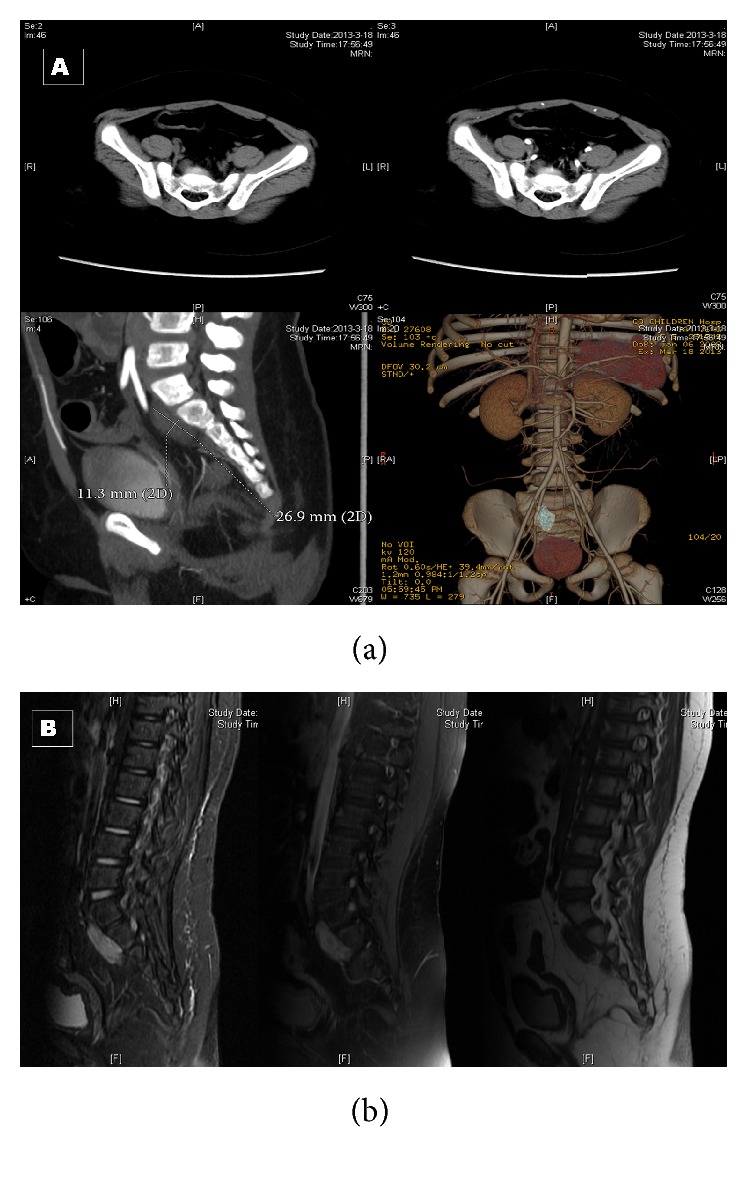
(a) The CT scan abdomen showed a dishomogeneous mass (11.2 mm × 26.9 mm × 11.3 mm) with obvious enhancement located before the sacral vertebra. (b) MR images revealing a presacral ganglioneuroma originated from sacral canal in S1-2.
